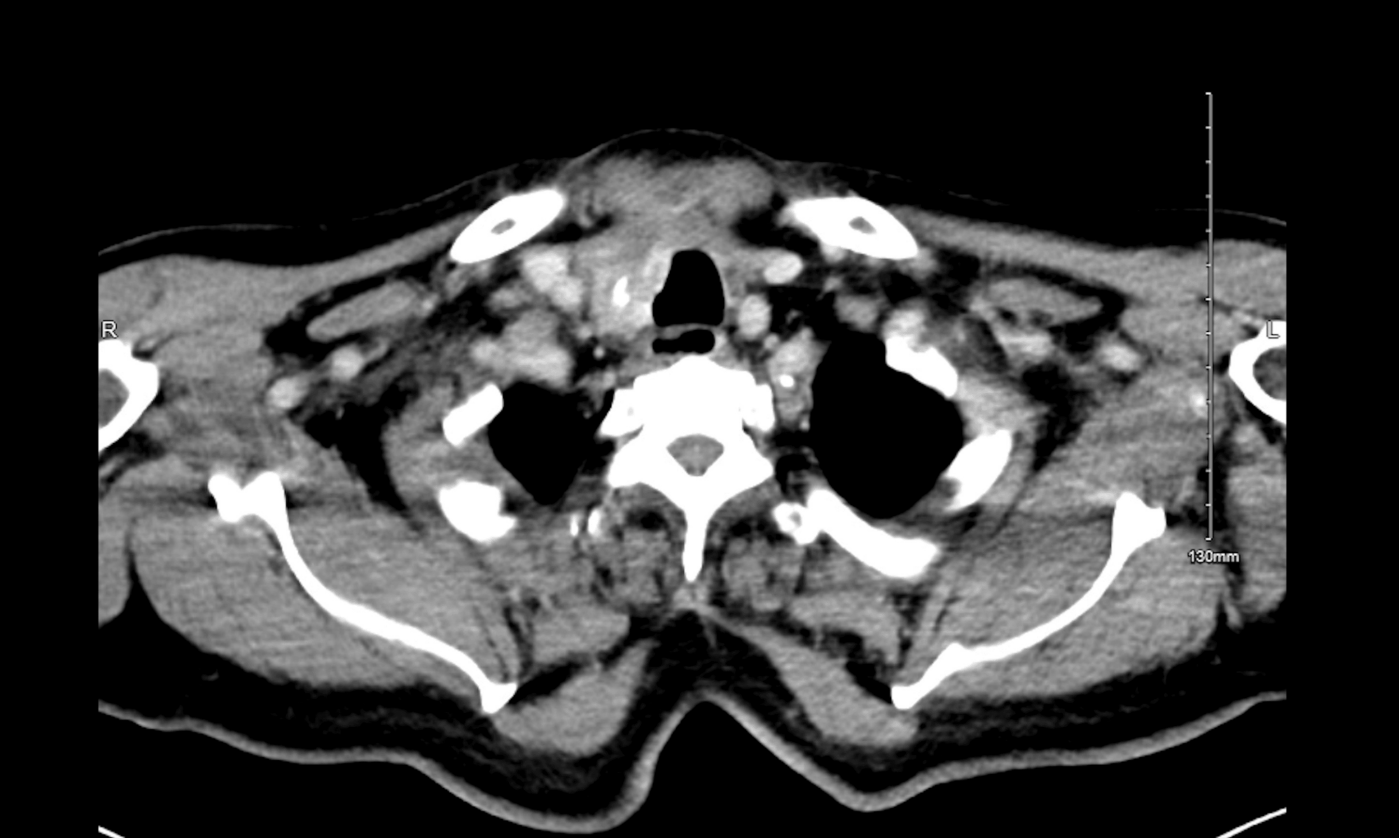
Staging CT scan. CT scan showing a cross-section of the neck with stable findings and no further metastasis.
